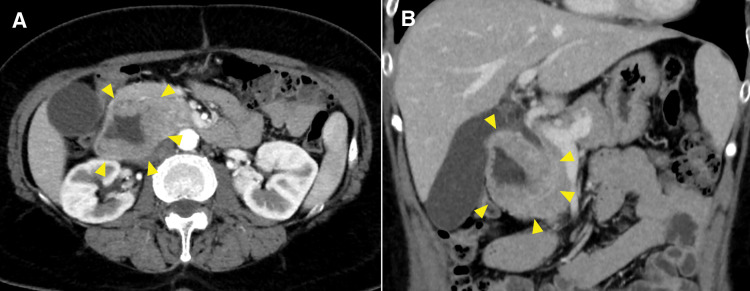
Contrast-enhanced CT findings. Contrast-enhanced CT shows a tumor in the second portion of the duodenum measuring 30 mm in diameter, with persistent enhancement and invasion of the pancreas (yellow arrowheads). (A) Axial view, arterial phase; (B) coronal view, portal phase.
